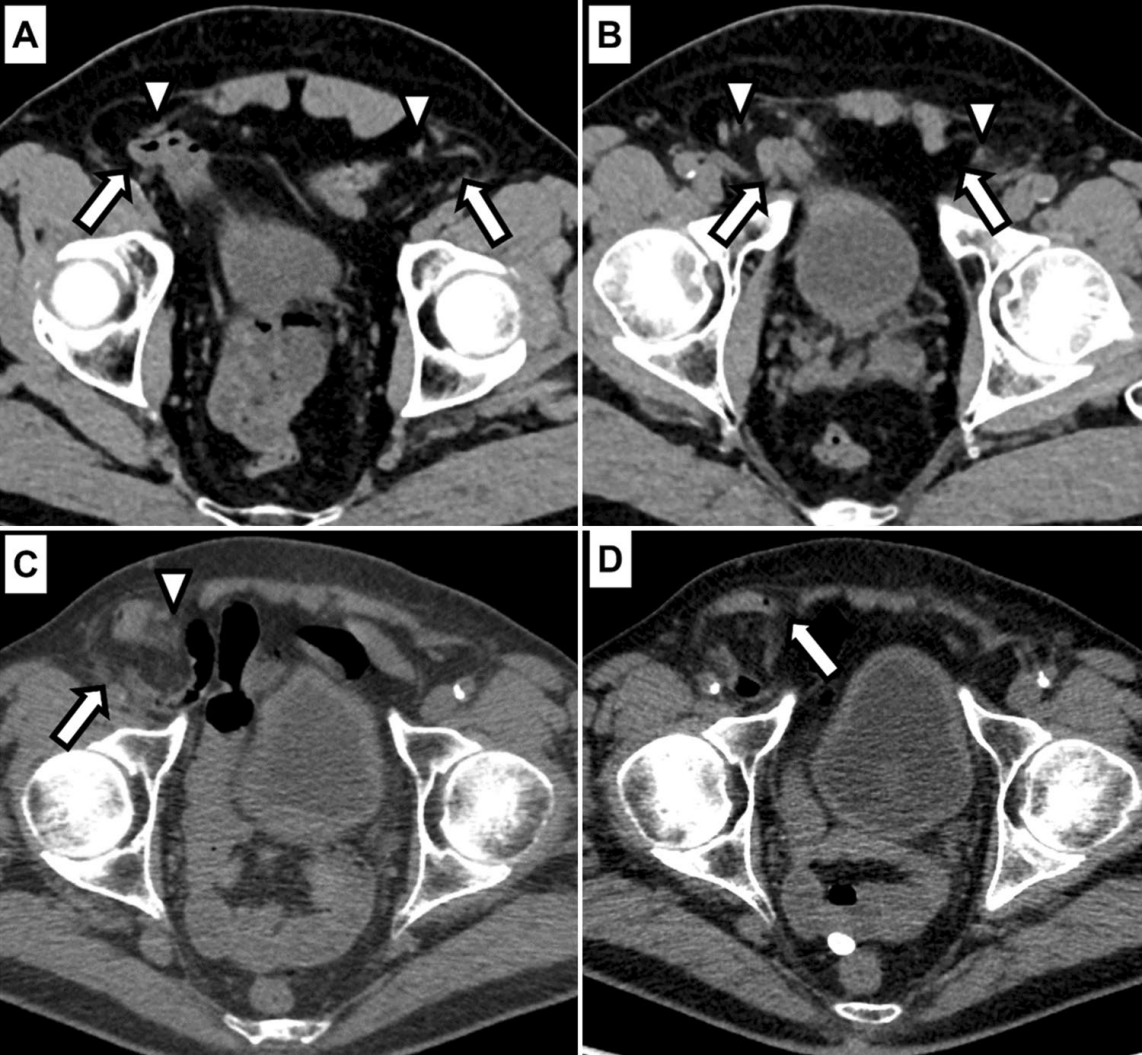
Two examples of combined inguinal hernias. Top row A, B shows axial CT images a few slices apart at the level of the groin in a patient with bilateral combined inguinal hernias. The indirect component (arrows in A) arises lateral to the inferior epigastric vessels (arrowhead in A) while the direct component (arrows in B) arises medial to the inferior epigastric vessels (arrowheads in B). This case was correctly classified by all three readers. Bottom row C, D shows axial CT images a few slices apart at the level of the groin in a patient with a right-sided combined inguinal hernia. The larger, indirect component (arrow in C) is seen lateral to the inferior epigastric vessels (arrowhead in C), but there is also a small direct component (arrow in D). This case was misclassified as indirect inguinal hernia by all three readers
